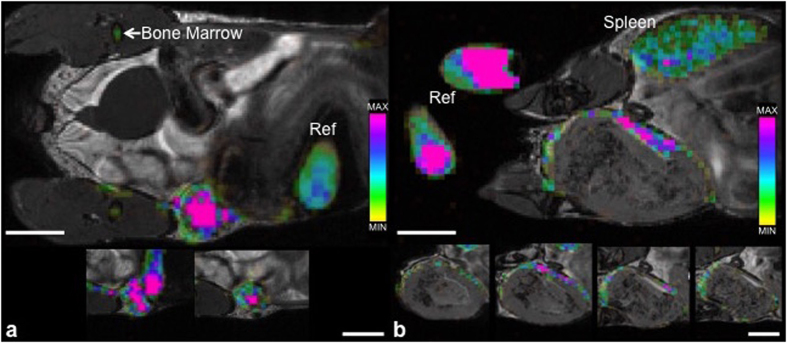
^19^F-based MRI cell tracking. bSSFP images of mammary fat pad tumors acquired 48 hours post PFC administration. (a) 4 days post 4T1 cancer cell injection: ^19^F signal is detected throughout the entire tumor with a higher density visualized within the center of the tumor (pink) when compared to the periphery. (b) 3 weeks post 4T1 cancer cell injection: ^19^F signal is detected, heterogeneous in density, only along the periphery of the tumor. Cropped images that are placed below each of the main images show the adjacent image slices (1 mm) containing ^19^F signal. The color bar demonstrates range of ^19^F spins. ^19^F signal is also detected in reference tubes (ref), bone marrow and spleen. Scale bars represent 5 mm.
